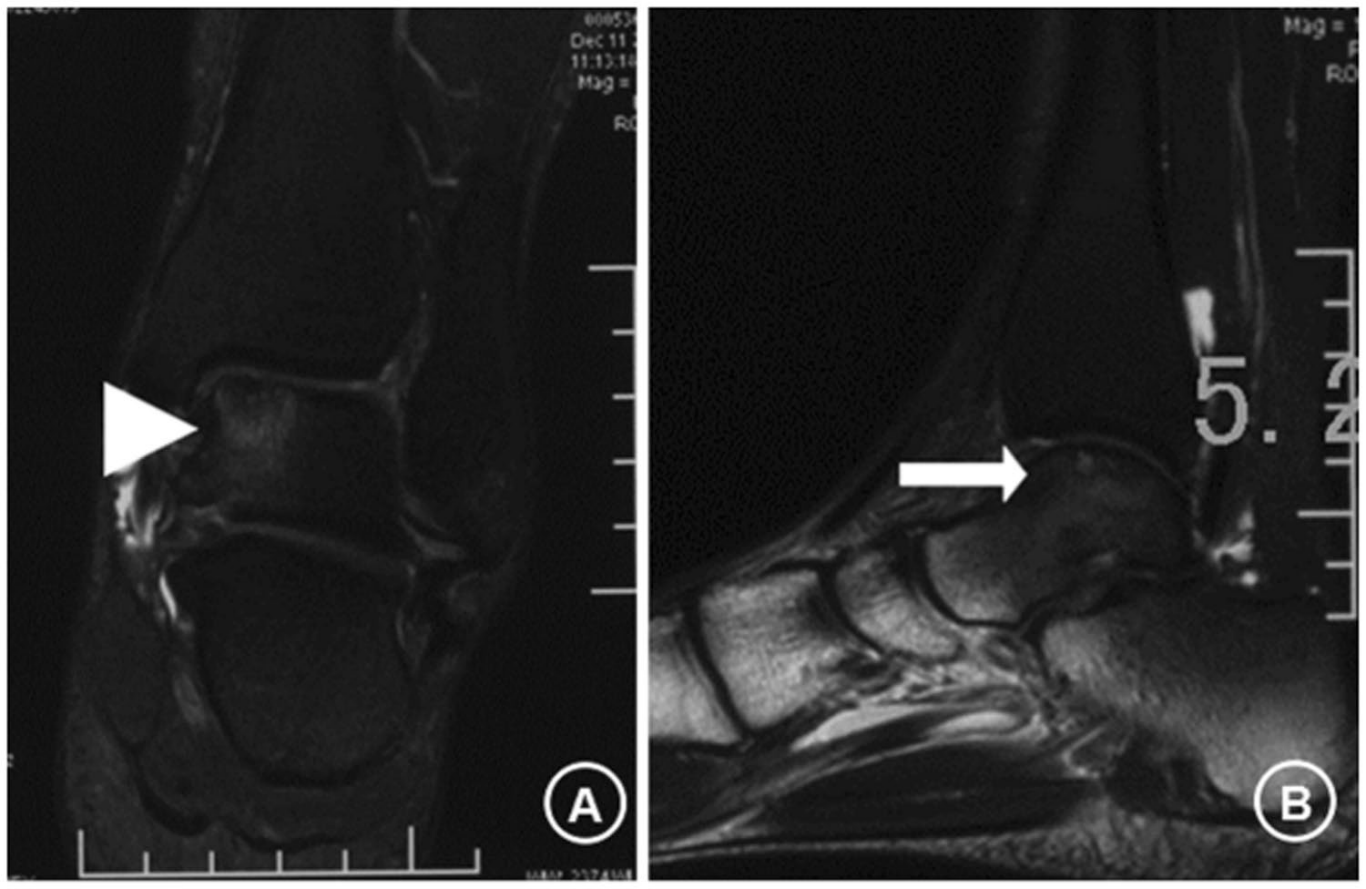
T2-weighted coronal (A) and sagittal (B) magnetic resonance images of the ankle. Images at 6 months post-retrograde autologous bone marrow cell transplantation via core drilling combined with focused extracorporeal shock wave treatment. Clear evidence of mild marrow edema and repair of osteochondral lesions (A, arrow head; B, arrow). Note: The patient consented to publish the specific information.
